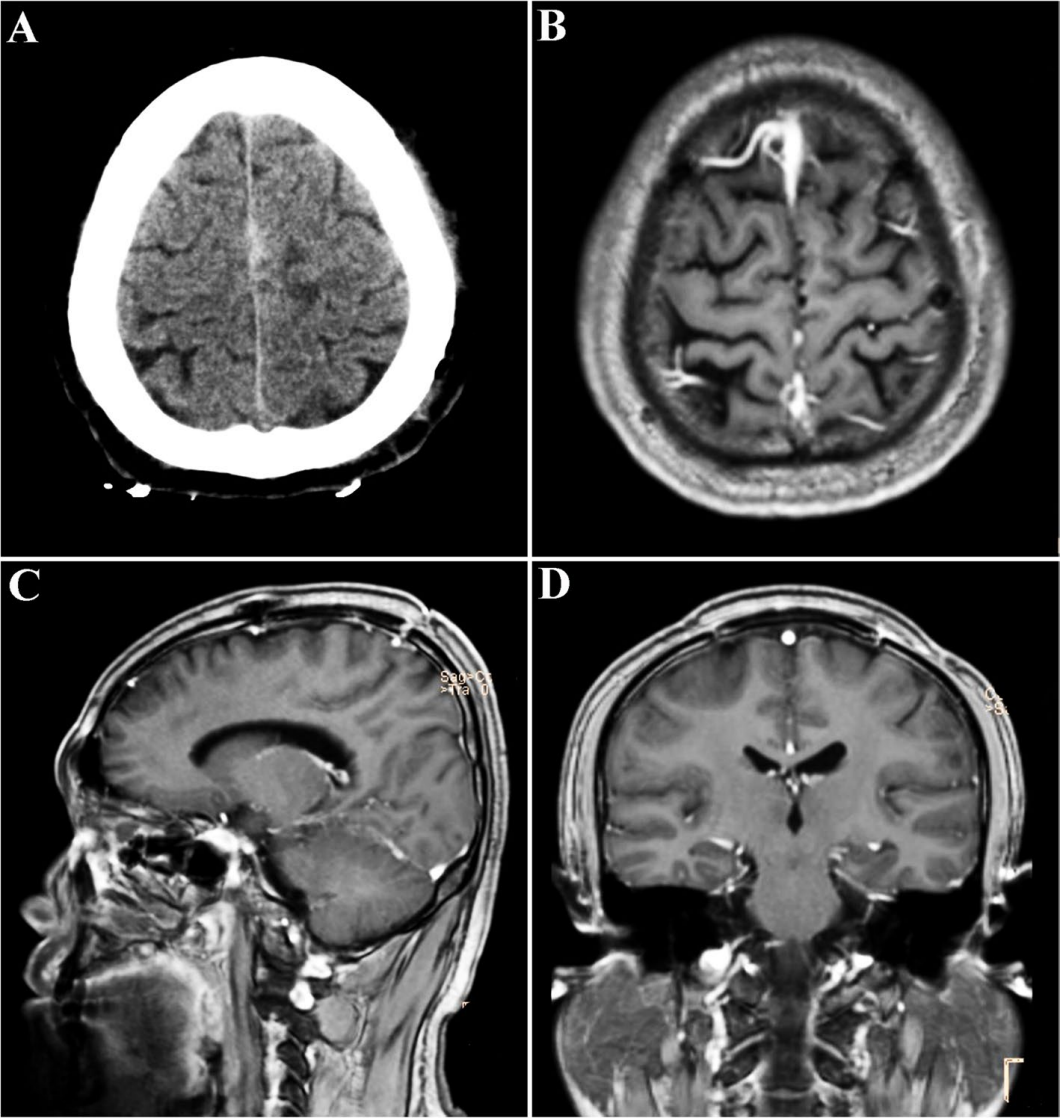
A Four hours after operation, the CT showed that the tumor was largely removed, and there was no obvious bleeding in the operation area. Head MRI at 9 months postoperative revealed no tumor recurrence. B Axial enhanced MRI 9 months after operation. C Sagittal enhanced MRI 9 months after operation. D Coronal enhanced MRI 9 months after operation
